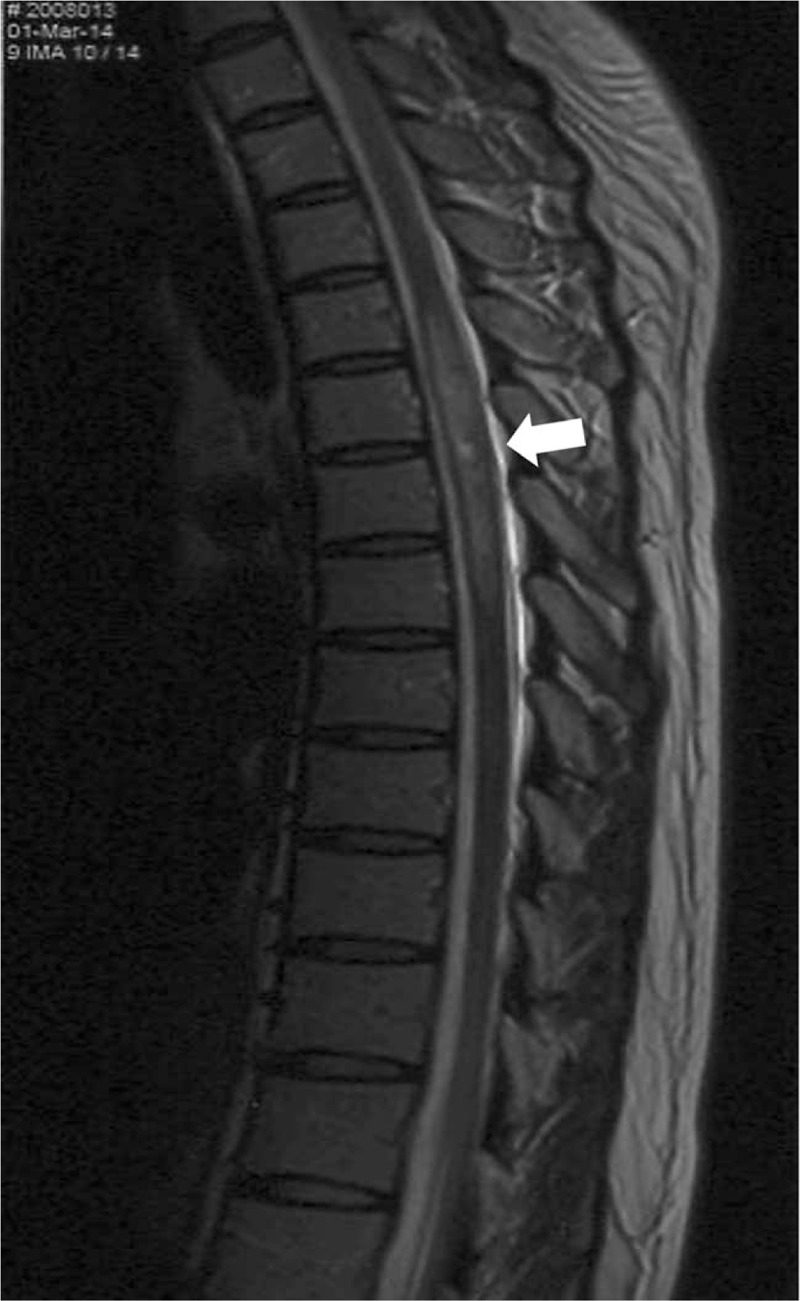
T2-weighted thoracic magnetic resonance image of the patient taken when transverse myelitis occurred. The arrow indicates high signal intensity in the spinal cord at the T5–8 level.
